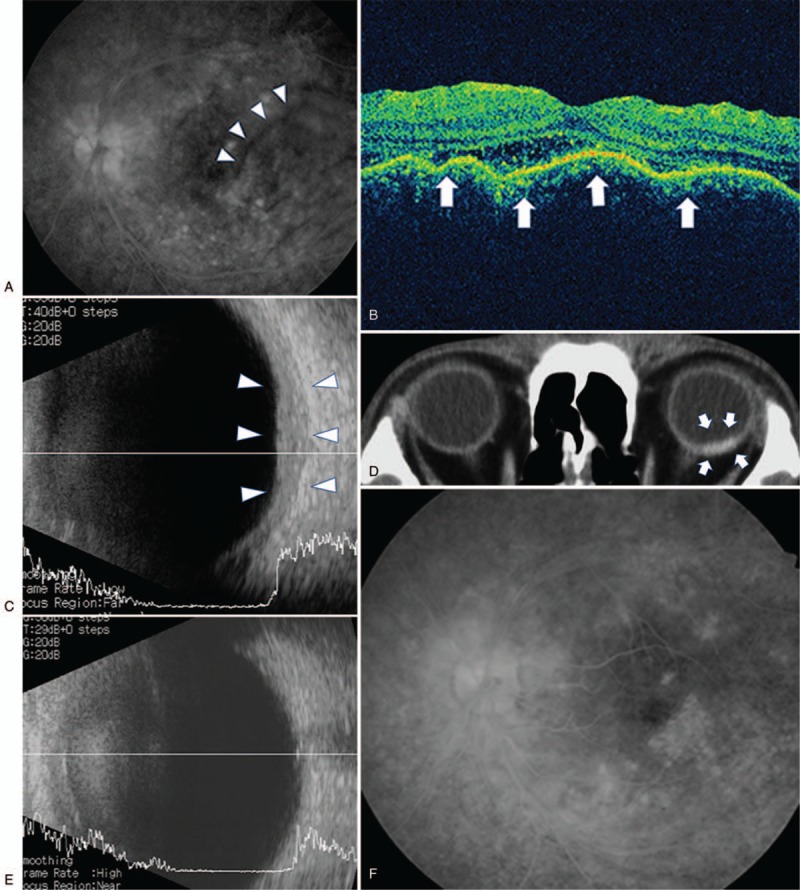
(A) Fluorescein angiography of the left eye showing peripheral vasculitis characteristic of Behcet disease and retinal folds at the posterior pole. (B) Optical coherence tomography showing choroidal folds (arrows) in the left eye. (C) B-scan ultrasonography of the left eye demonstrating thickened sclera (arrowheads). (D) CT scan of the patient showing contrast enhancement (arrows) localized to the posterior sclera of the left eye. (E) B-scan ultrasonography of the left eye 43 weeks after treatment showing disappearance of choroid hypertrophy. (F) Fluorescein angiography of the left eye 43 weeks after treatment showing disappearance of retinal folds.
